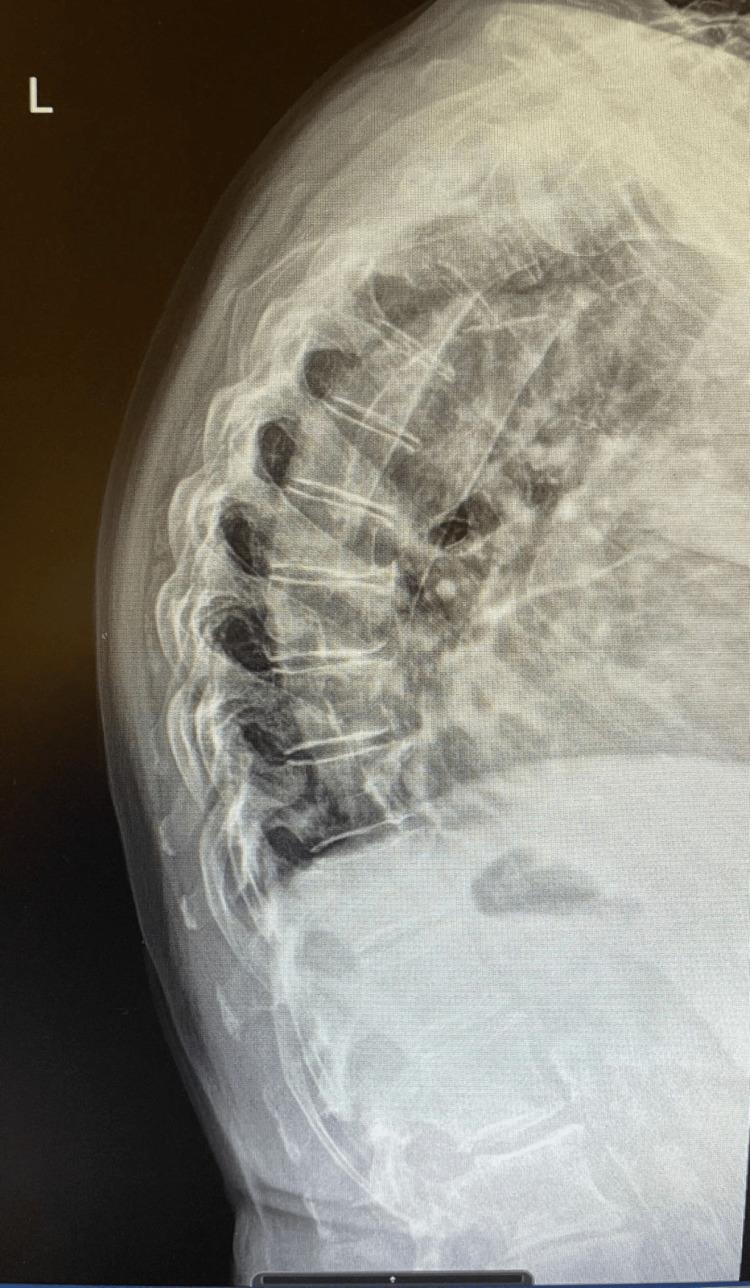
Thoracic spine X-ray lateral view Lateral view thoracic spine X-ray shows mild to moderate generalised degenerative changes with no evidence of vertebral collapse.
